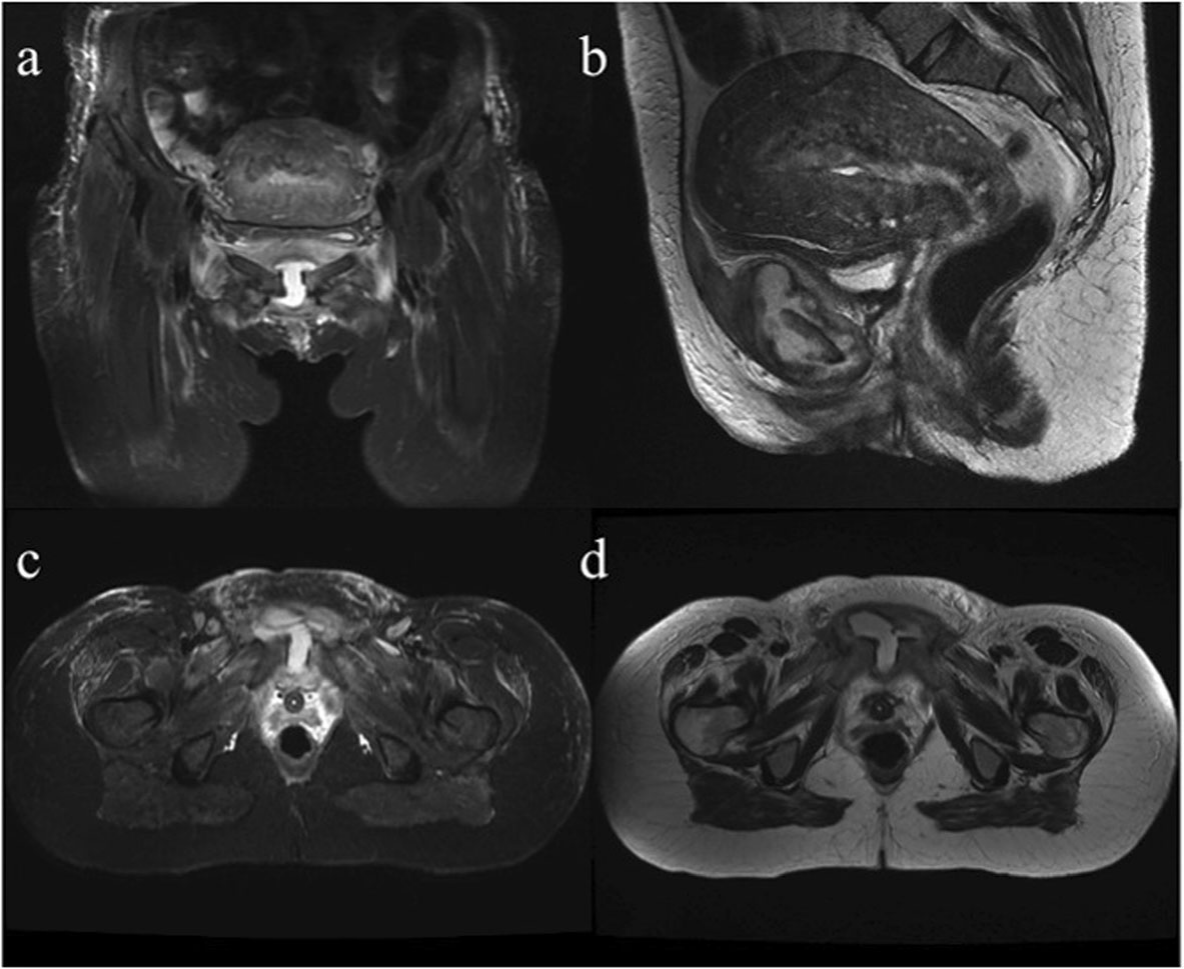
Pelvic magnetic resonance performed at day 8. Coronal short TI inversion recovery (STIR) (a), sagittal T2-weighted (b), axial STIR (c), and axial T2-weighted (d) images show pubic symphysis enlargement, abundant joint effusion with synovial thickening forming a pseudo-capsulated fluid collection within the symphysis, severe bone edema involving both pubic branches and edematous subcutaneous tissues
